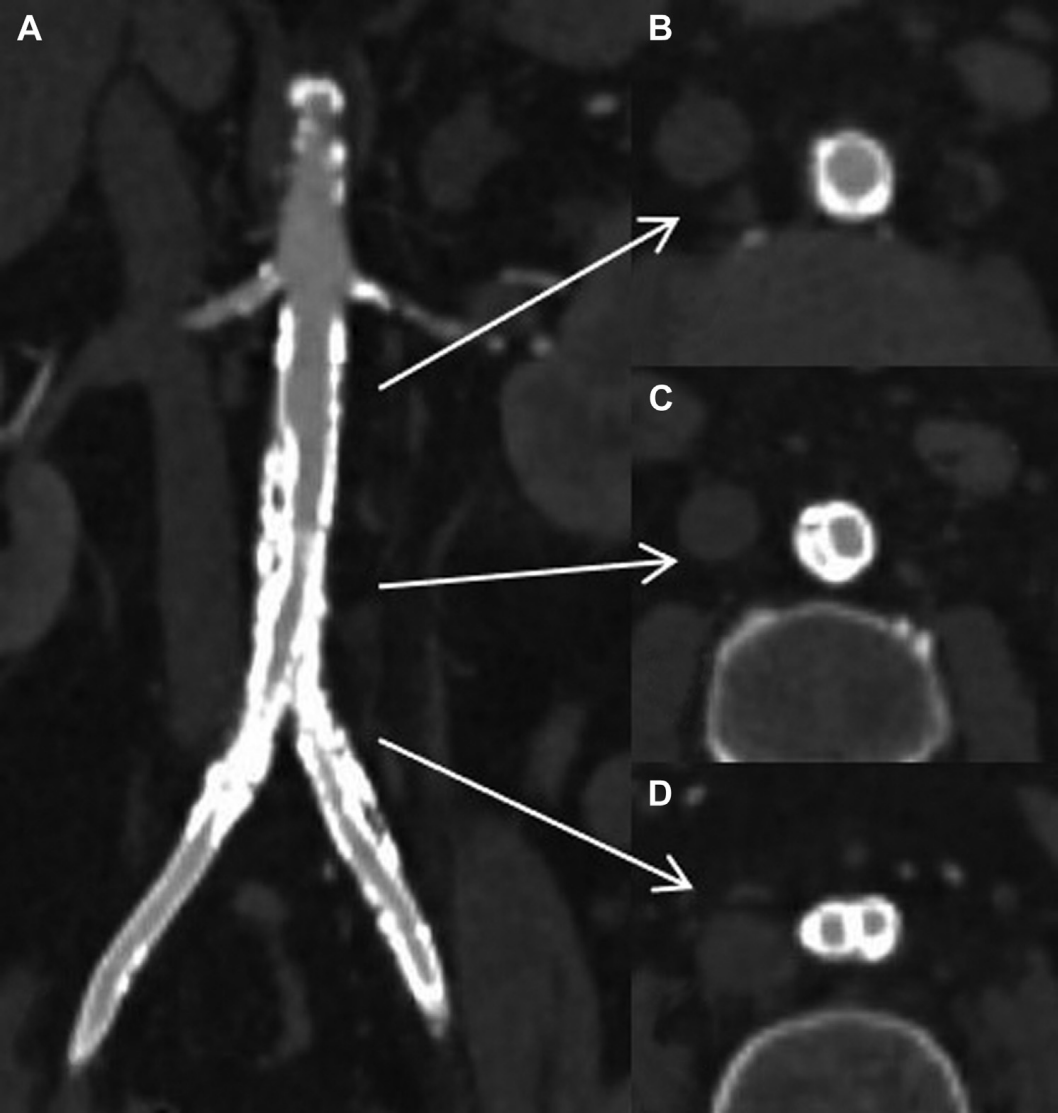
A, Reconstruction of postoperative computed tomography angiography (CTA) showing fracture of the calcified lesion at the mid-infrarenal aorta (B) and regular patency of the covered endovascular reconstruction of aortic bifurcation (C-D).
